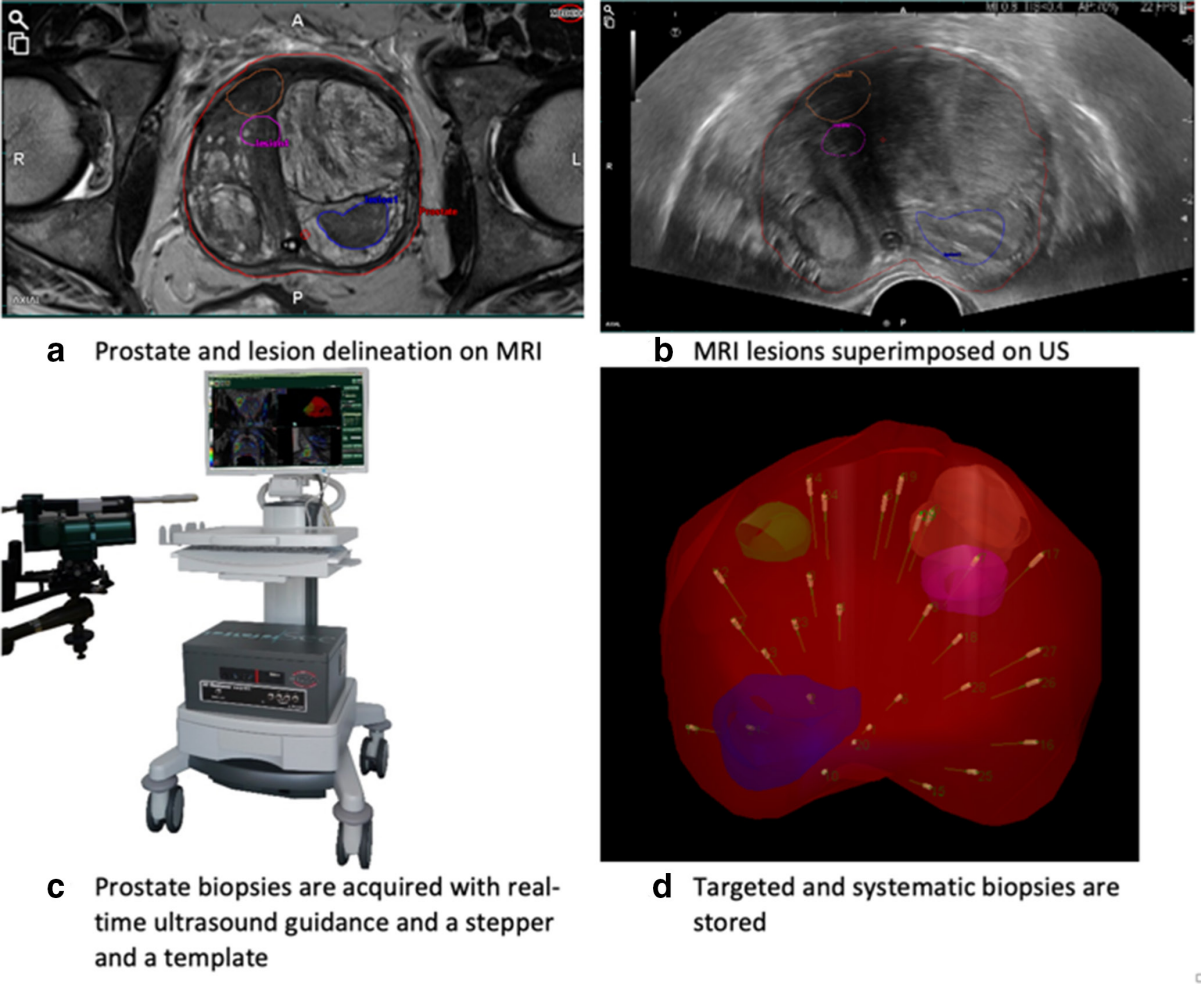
Example of an MRI fusion template-guided transperineal biopsy technique (BiopSeeTM, Medcom).
